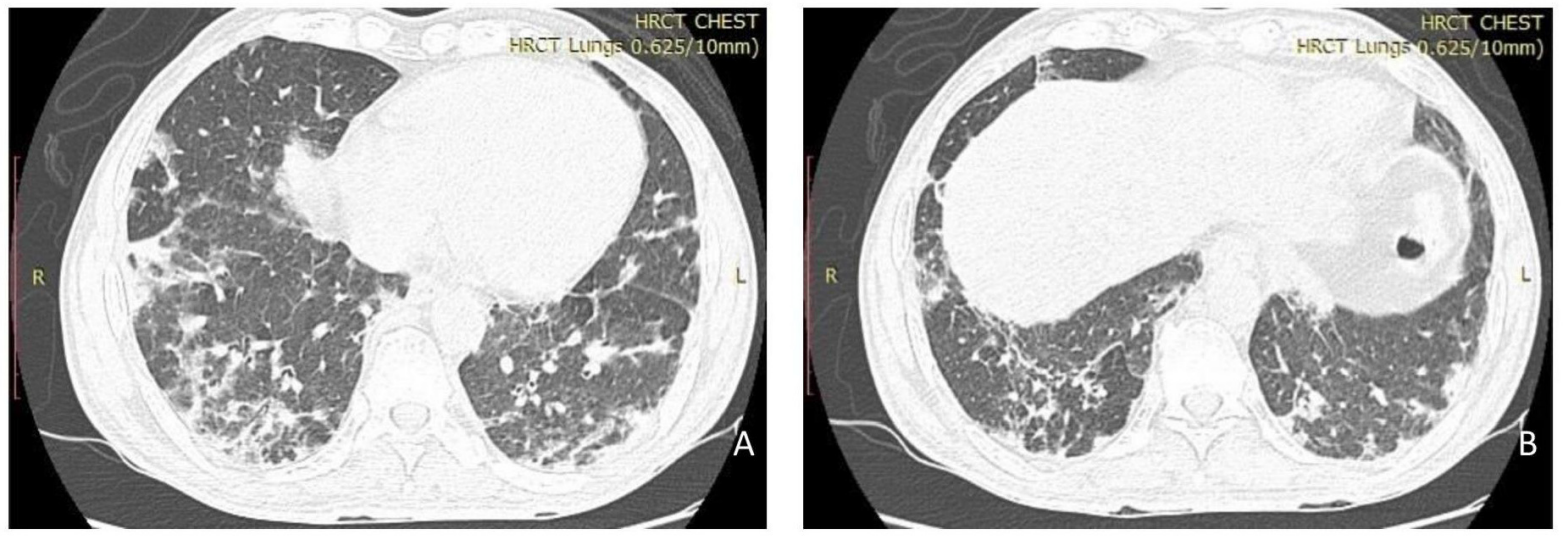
HRCT lungs- Bilateral peripheral lower lobe predominant reticulation with patchy consolidation and ground glass changes.
